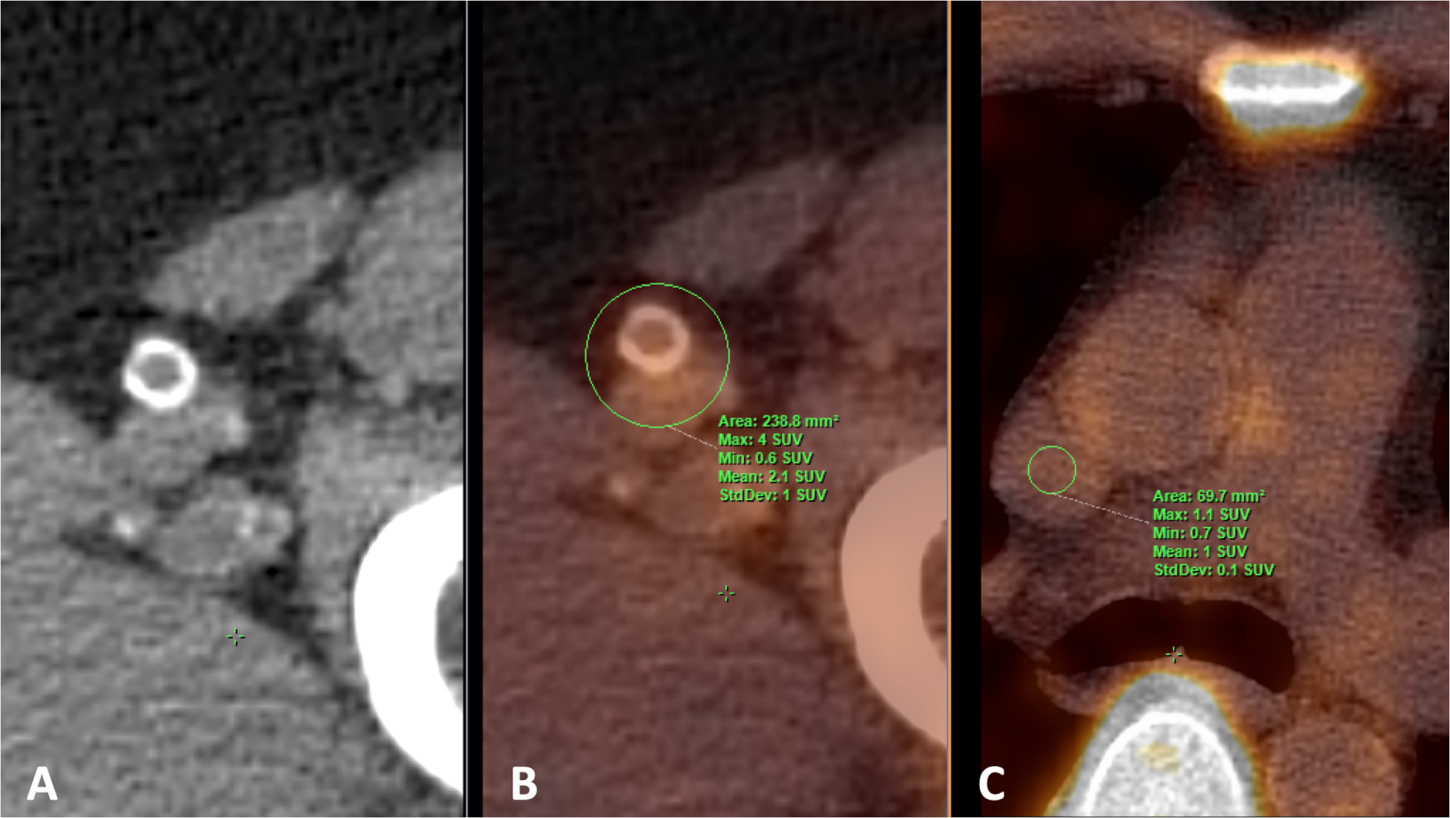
Axial CT image A showing circular calcification in the superficial femoral artery and fusion image B with a circular region of interest (ROI) drawn for quantification ^18^F-NaF SUVmax in the femoral artery. Axial fusion image (C) demonstrating background measurement of ^18^F-NaF SUVmean in the superior vena cava using a circular ROI
